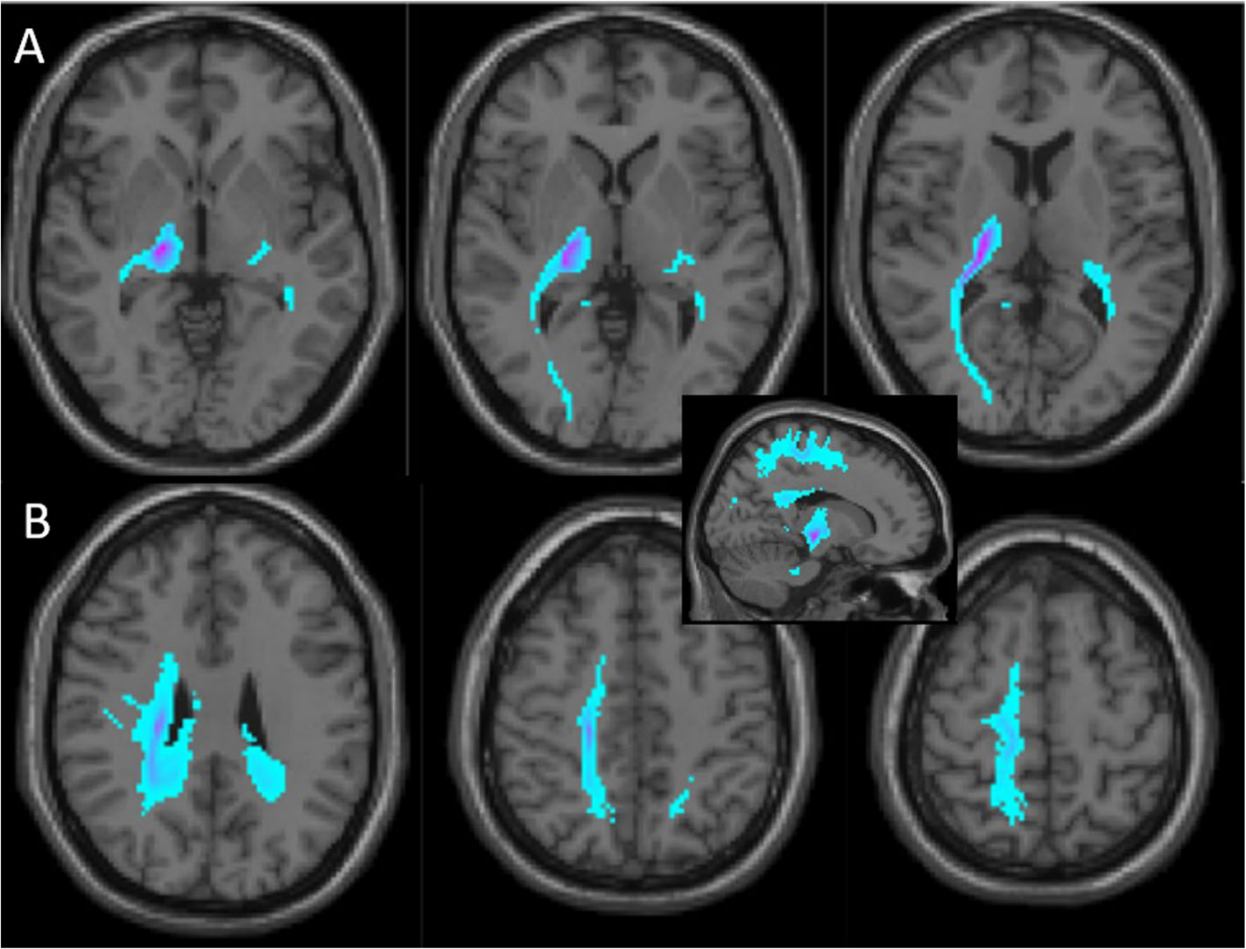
Probabilistic connectivity of the right thalamic lesion based on Magnetic Resonance Imaging (Diffusion Tensor Imaging). This analysis is overlayed on the same T1 brain image as in the Fig. 1c. Blue clusters disclose areas to which the lesion was expected to be connected (darker color indicates stronger connectivity), independently from the actual number of residual fibers. a Successive sections from the lesion bottomward show connectivity with the ipsilateral occipital region. b In more superficial sections, lesion connectivity points to a superficial linear paramedial area spanning from the ipsilateral frontal cortex through the superior parietal lobule, including also the primary somatosensory cortex (middle and most right panels). More deeply and internally, the inferior and anterior precuneus regions seem to be involved (most left panel). The inset corresponds to a mesial view further confirming the antero-posterior extension of the most superficial (frontal, parietal cortices and superior parietal lobule) and the deepest (precuneus) connections of the thalamic lesion
